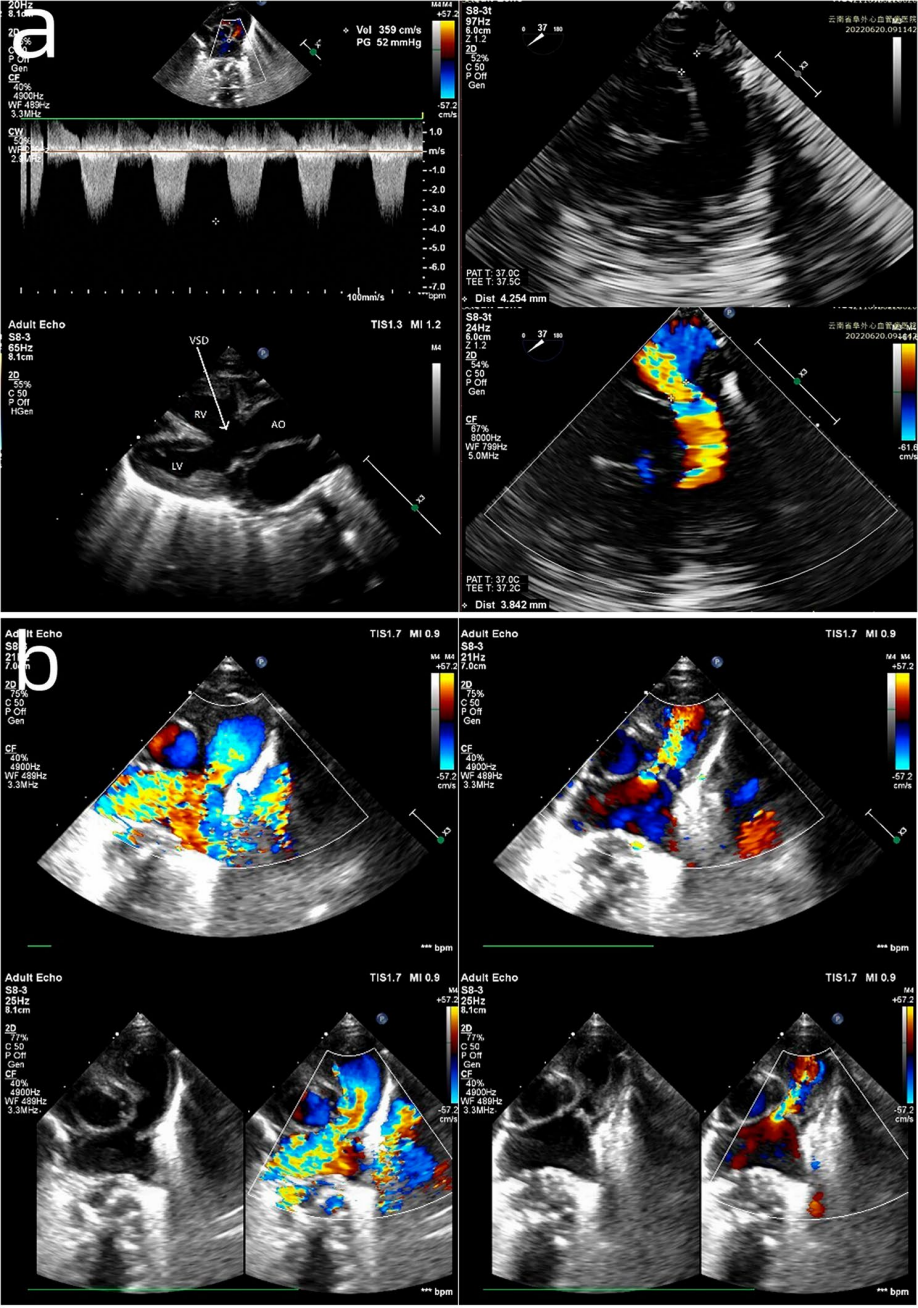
Preoperative ultrasound showed massive pulmonary artery regurgitation (a), stenosis and aneurysmal dilatation of the main pulmonary artery (b), ventricular septal defect (VSD), and aorta (AO) overriding. LV, left ventricle; RV, right ventricle
